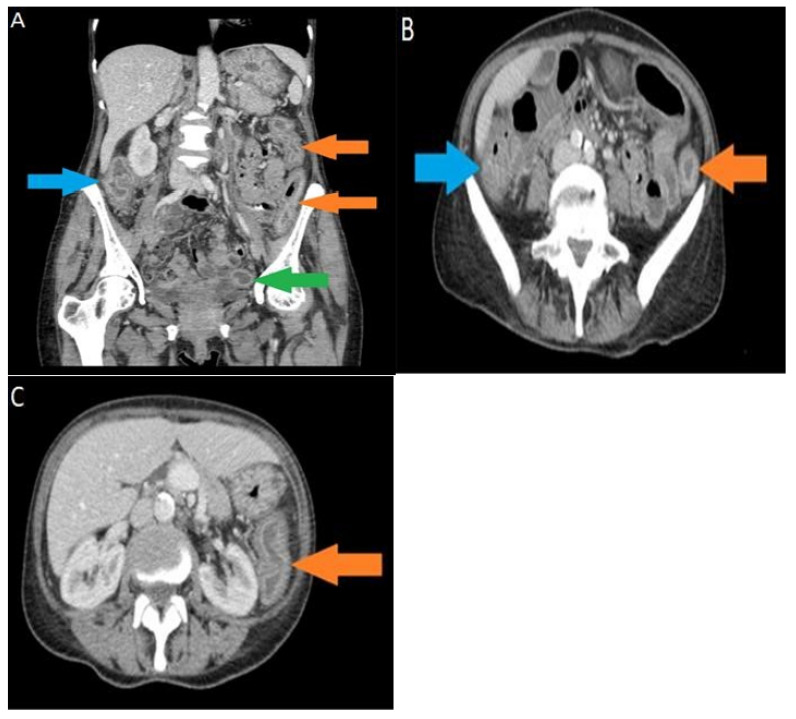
(A) Selected coronal CT image, acquired in the portal venous phase, shows marked bowel wall thickening and mucosal hyperenhancement in the ascending colon (blue arrow), descending colon (orange arrows), and sigmoid colon (green arrow), in agreement with the diagnosis of active multifocal colitis; (B) selected axial CT image, acquired in the portal venous phase, shows marked bowel wall thickening and mucosal hyperenhancement in the hepatic flexure of the large bowel (blue arrow) and splenic flexure of the large bowel (orange arrow), in agreement with the diagnosis of active multifocal colitis; (C) selected axial CT image, acquired in the portal venous phase, shows marked bowel wall thickening and mucosal hyperenhancement in the descending colon (orange arrow), in agreement with the diagnosis of active colitis.
